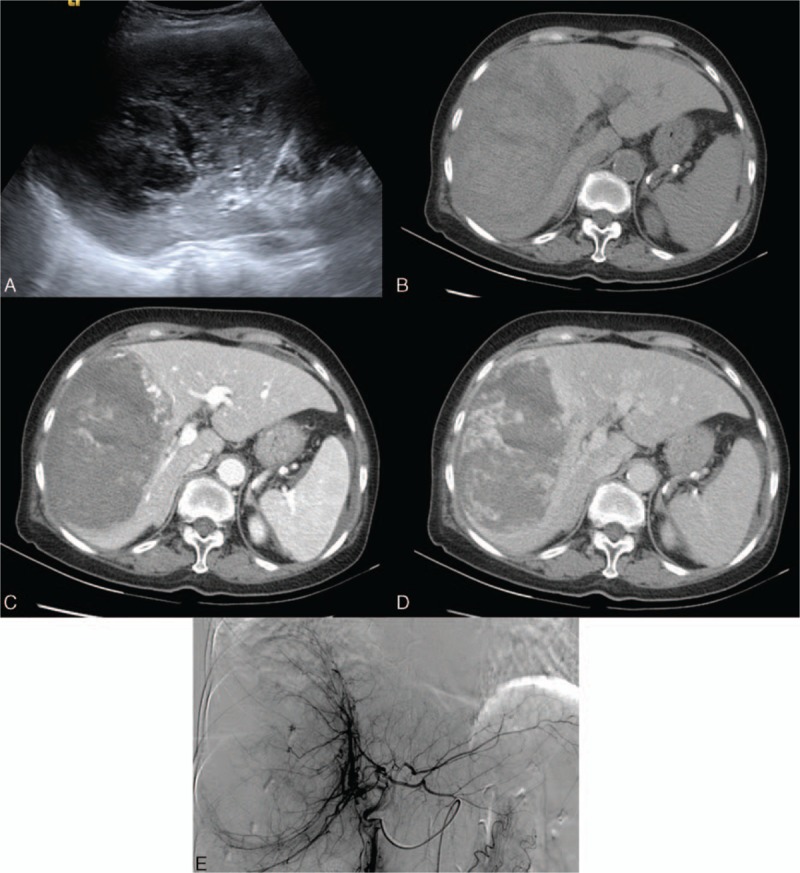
An 80-year-old woman with an angiosarcoma. A, A heterogeneously isoechoic mass with internal hypoechoic necrotic areas on sonography. B, The tumor mass has hypodense areas, indicating necrosis. C, A bizarrely shaped peripheral arterial enhancement is characteristic of angiosarcoma. D, Focally progressive but incomplete enhancement of the tumor is observed on the later phase. E, Hypervascularity with prominent vascular channels is depicted on celiac angiography.
